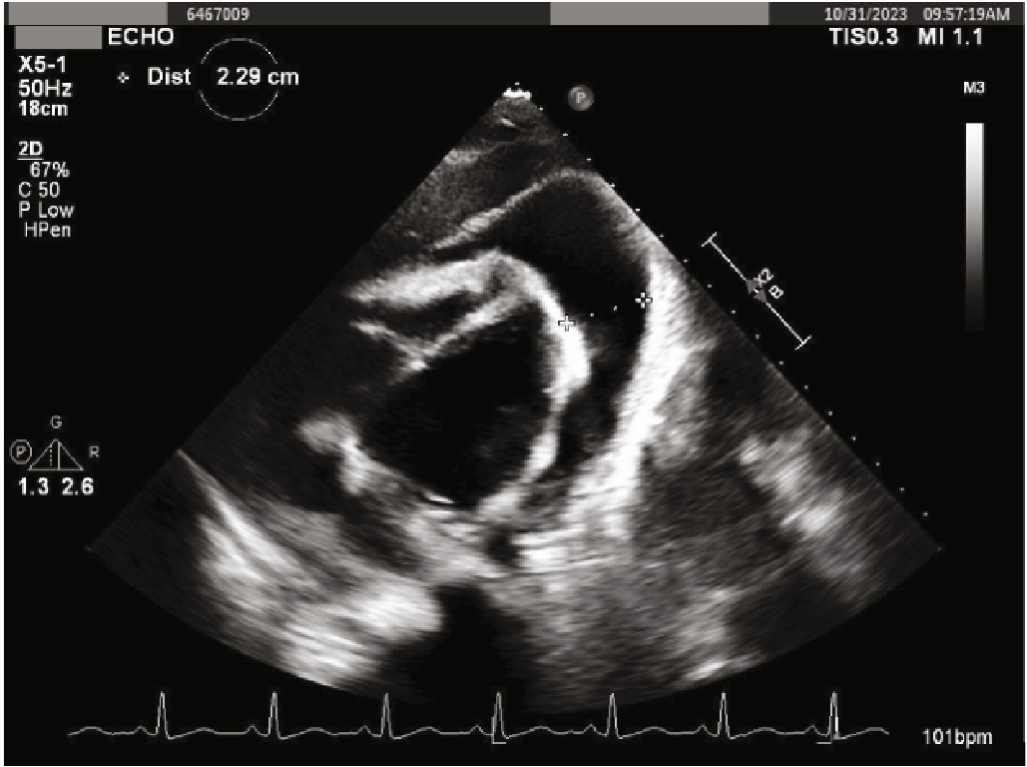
Outpatient echocardiogram demonstrating a 16-cm mass.
